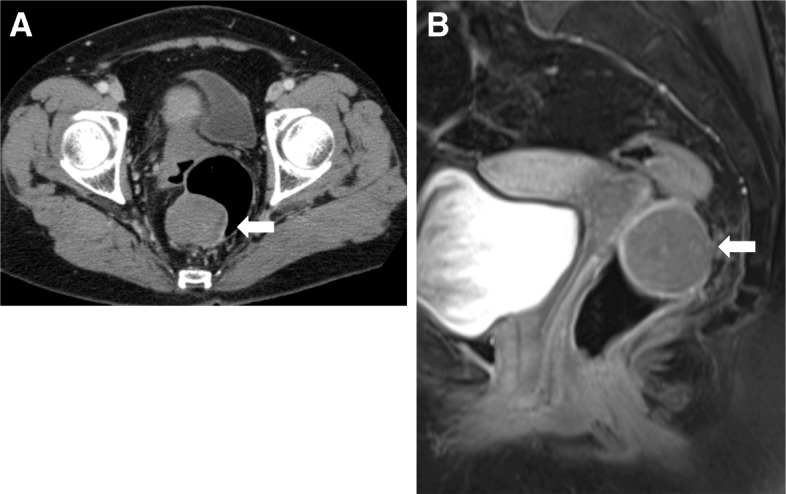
CT and MRI examinations. a CT scan of pelvis showing tumor 4.5 cm in diameter (white arrowhead). b MRI showing tumor at middle rectum (white arrowhead)
